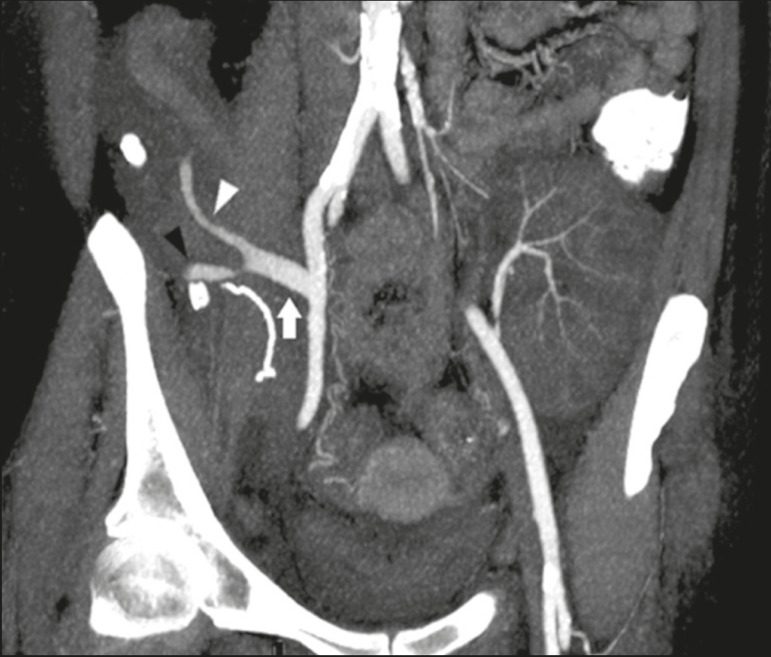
Maximum intensity projection image in the arterial phase of contrast-enhanced CT (coronal reconstruction), showing a patent Y graft (white arrow) anastomosed to the right external iliac artery. Note the anastomosis of the Y graft to the branches of the splenic artery (white arrowhead) and superior mesenteric artery (black arrowhead), with narrowing of the lumen of the superior mesenteric artery.
